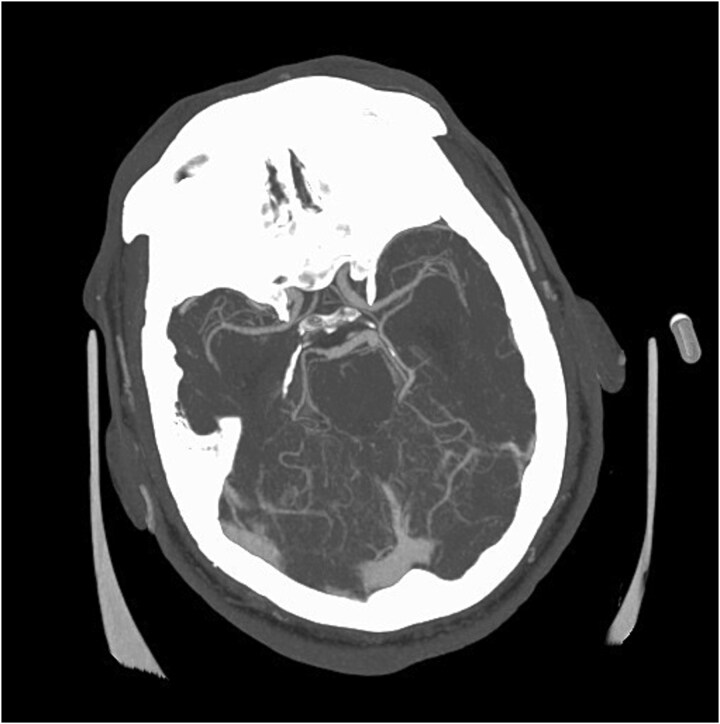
Repeat CTA head demonstrating new multifocal intracranial vessels stenosis.
